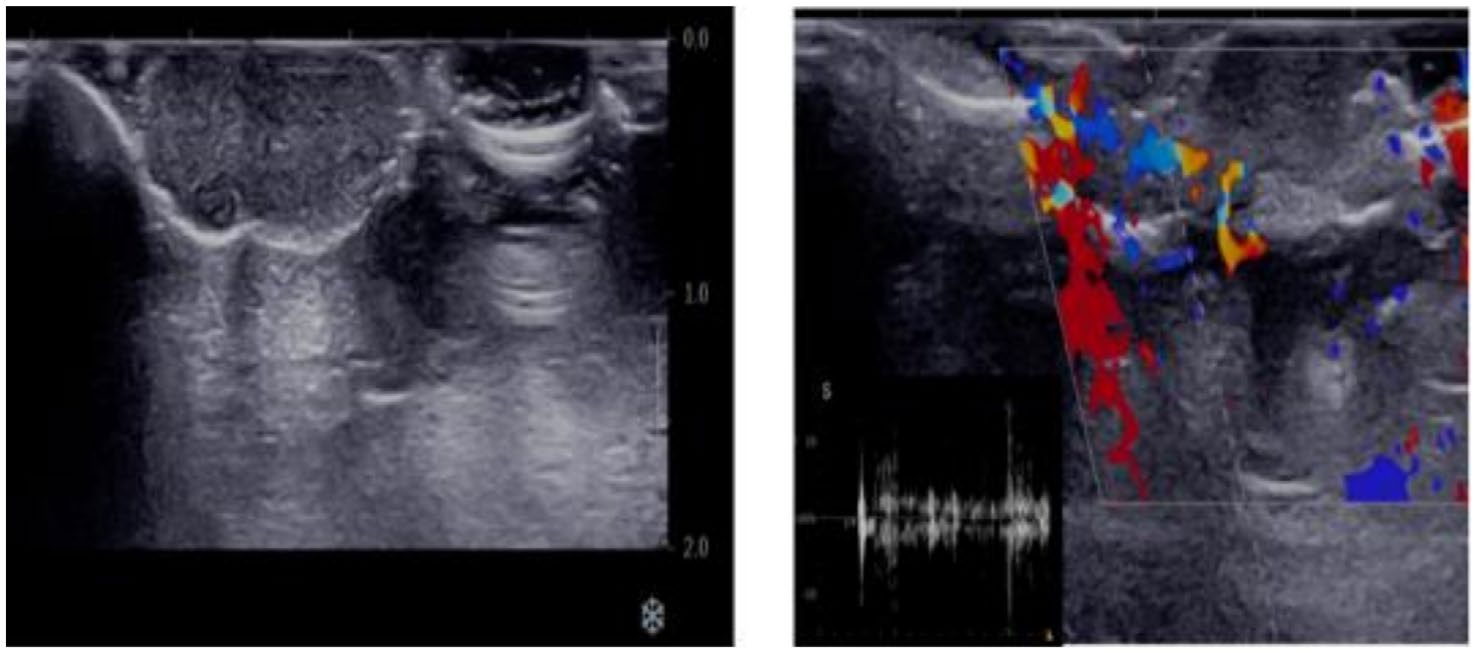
Color Doppler ultrasound using an 18 MHz linear probe revealed a well‐defined hypoechoic pedunculated mass measuring 18 × 18 mm in the vulva, exhibiting mild peripheral and internal vascularity with a peak systolic velocity of approximately 10 cm/s.
